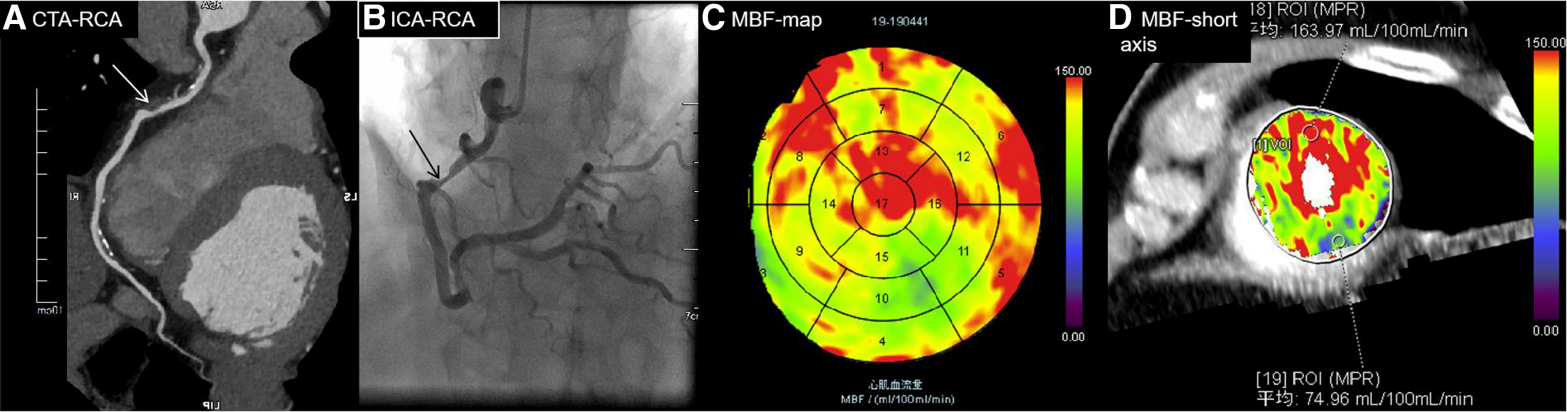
A 70-year-old man diagnosed with hypertension and diabetes for 7 years presented with chest pain. (A) CTA showing moderate stenosis of 75% in the middle RCA (white arrow), indicating MI. (B) ICA showing severe stenosis of 80% in the middle of the RCA (black arrow), with an FFR value of 0.7, indicating MI. (C) MBF map image showing decreased perfusion in the RCA territory (blue and green areas in segments 10, 11, and 15). (D) MBF short-axis image showing decreased perfusion in the inferior wall of the left ventricle, specifically at region of interest (ROI) 19. The MBFa value is 74.96 ml/100 ml/min, and the MBF-ratio is 0.46 (74.96/163.97) (highest MBF, ROI 18). The MBFa and MBF-ratio values were consistent with FFR.RCA, right coronary artery.
